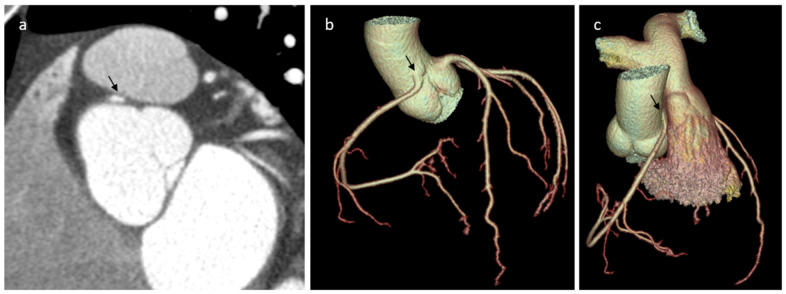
Right coronary artery high takeoff between the right and left coronary sinuses with interarterial course in axial (a) and volume-rendering (b,c) computed tomography images (arrows).
